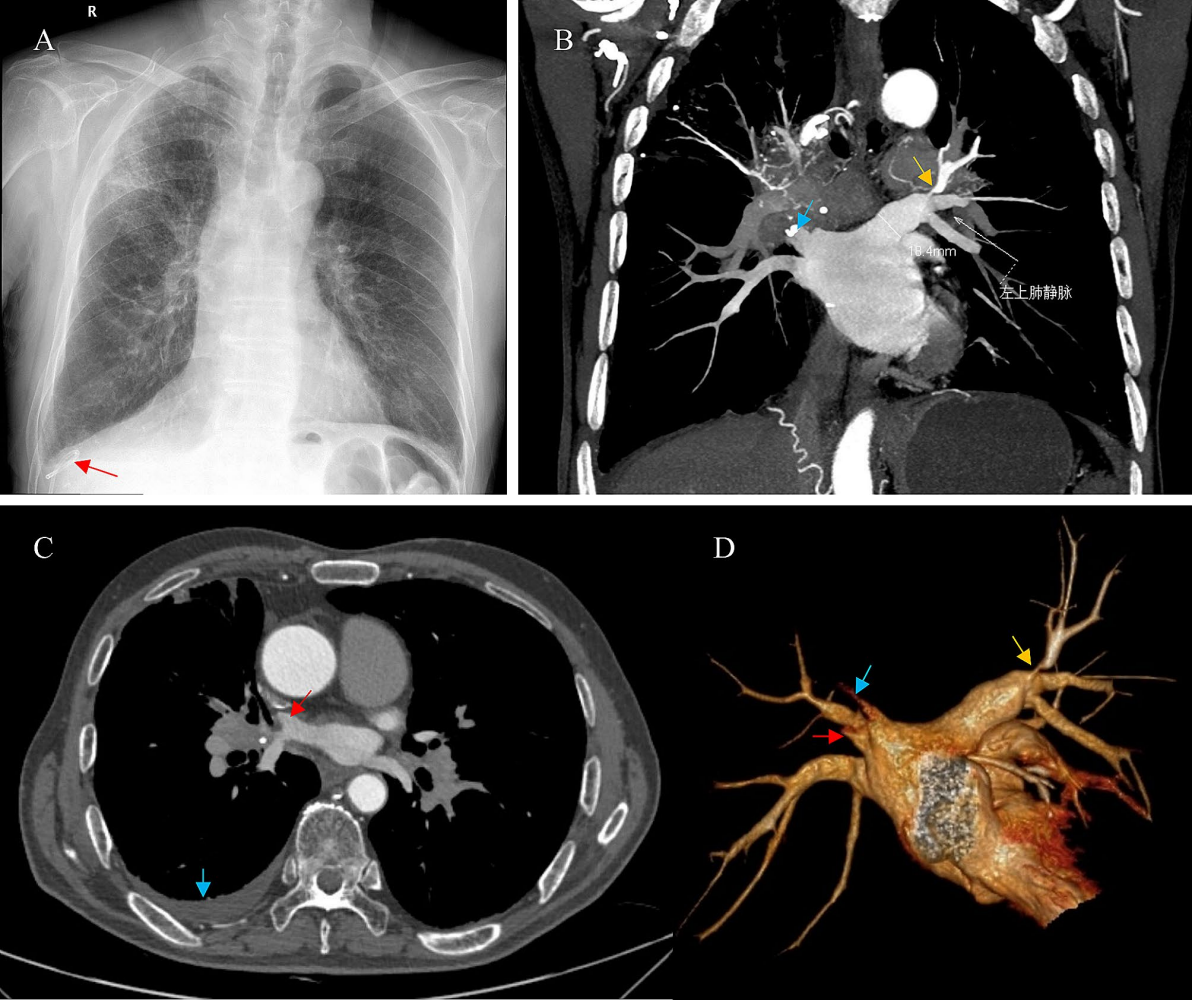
A, Chest X-ray image showing the drainage tube in the right lung (red arrow). B, Enhanced chest CT: The coronal view shows LSPV stenosis (yellow arrow) and RSPV occlusion (blue arrow). C, Enhanced chest CT: Axial view showing RSPV occlusion (red arrow) and pleural effusion (blue arrow). D, Virtual reconstructed CT image showing LSPV stenosis (yellow arrow) and RSPV occlusion (blue arrow, V1; red arrow, V2)
